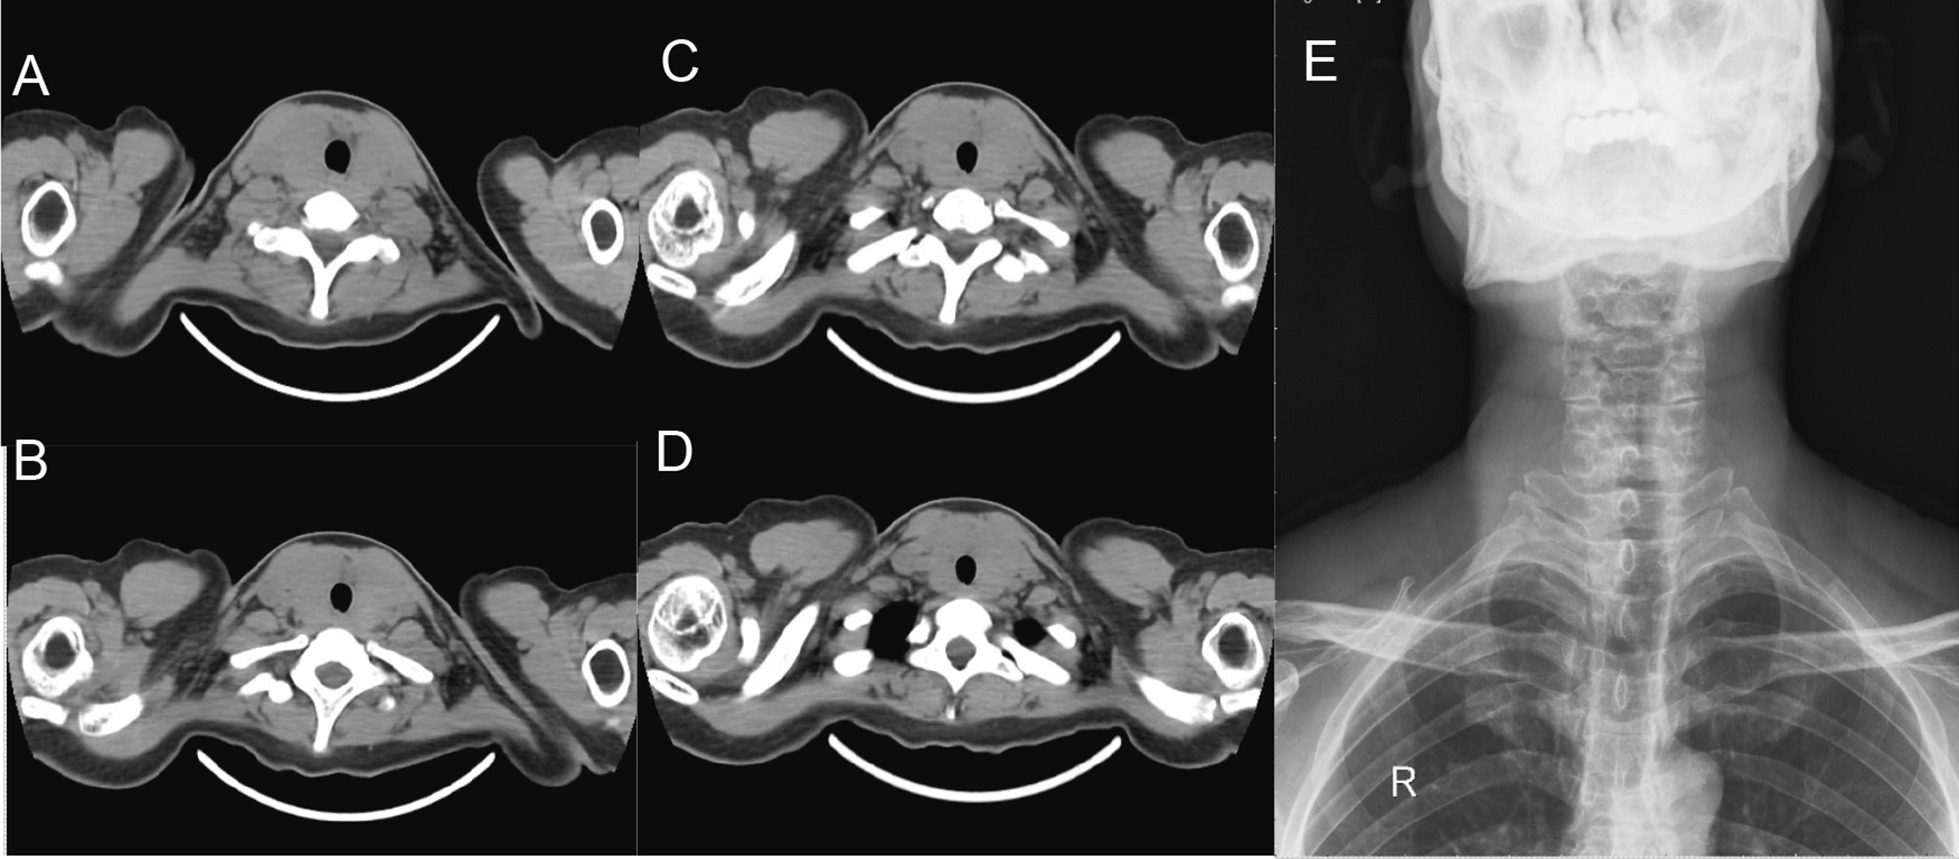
Characteristic imaging findings of IgG4-related thyroiditis. A–D represented increased thyroid volume with decreased density in CT images of 4 IgG4-related thyroiditis patients. E Showed tracheal compression due to increased thyroid volume
